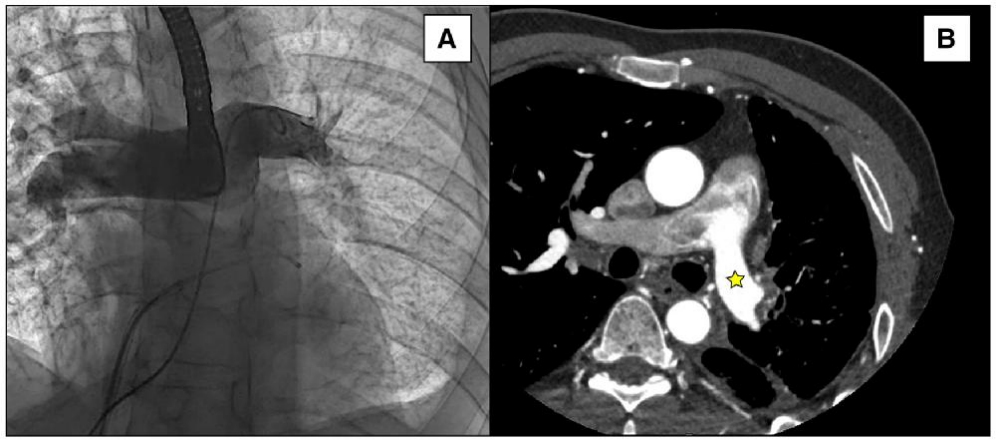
(*A*) Pulmonary artery angiography (under transoesophageal ultrasonography guidance) showing absence of contrast agent flow in the distal part of the left pulmonary artery. (*B*) Computed tomography scan showing stagnation of contrast agent in the left pulmonary artery (star).
